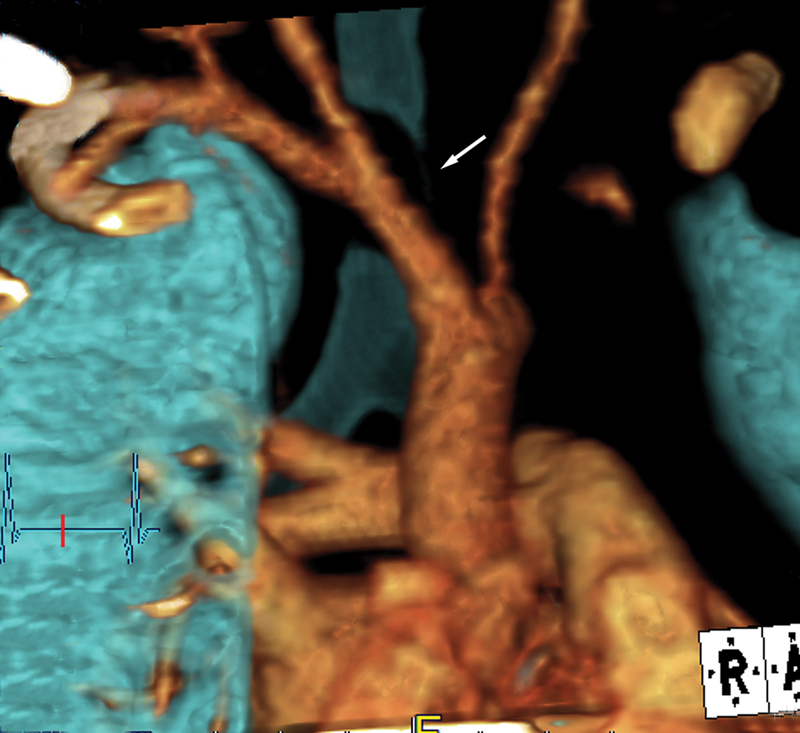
Three-dimensional volume rendered computed tomography image of the trachea demonstrates the innominate artery compressing the right lateral wall of the trachea (arrow).
